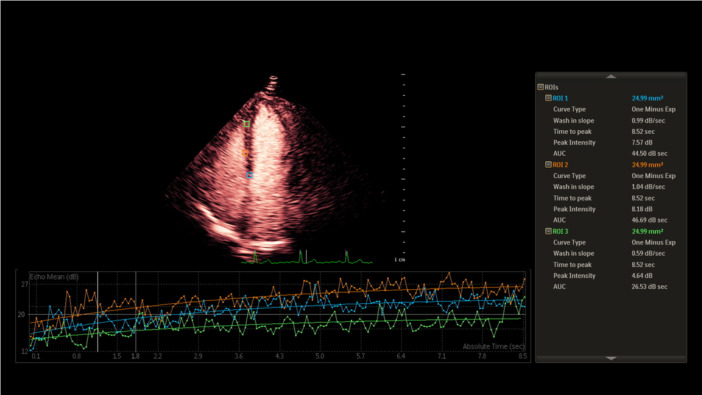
Quantitative Myocardial Contrast Echocardiography (MCE) Analysis in Apical Four‐Chamber View. AUC, area under the curve; ROI, regions of interest.
